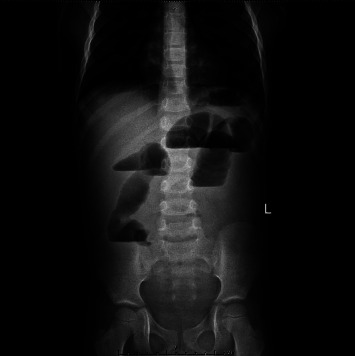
Abdominal X-ray. X-ray showing multiple air-fluid levels, which is suggestive of bowel obstruction.
